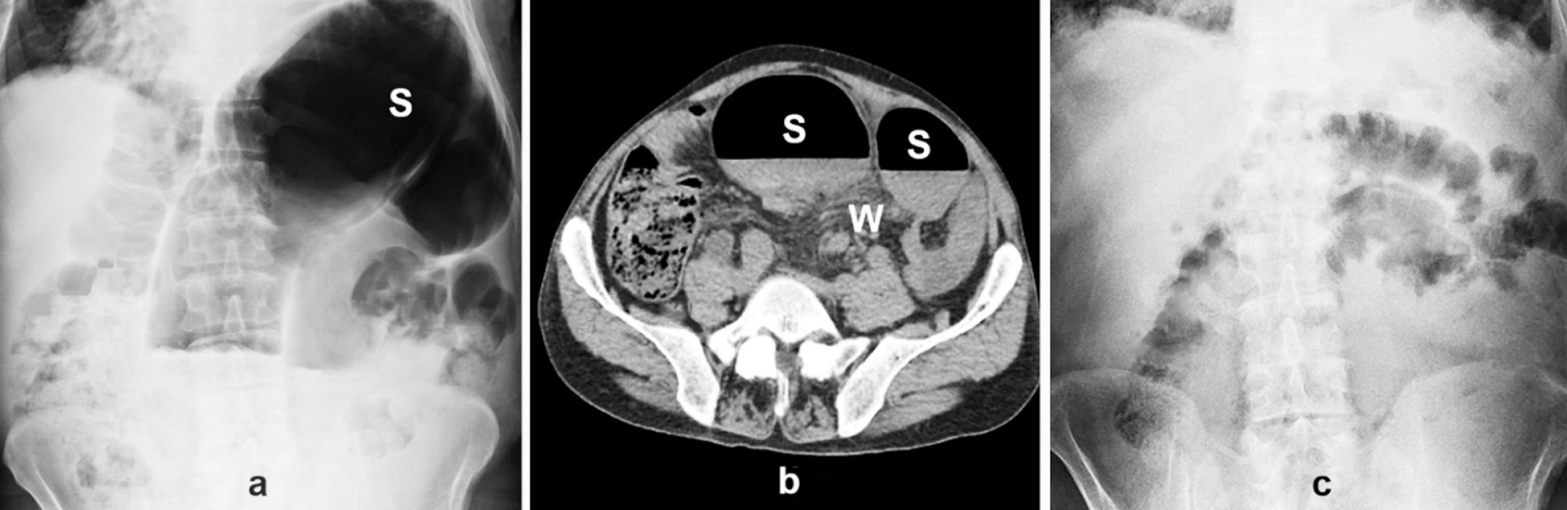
a) Abdominal X-ray radiography image (S: Dilated sigmoid colon) b) Axial abdominal computerized tomography image (S: Dilated sigmoid colon, W: Mesenteric whirl sign) c) Control abdominal X-ray radiography image.
